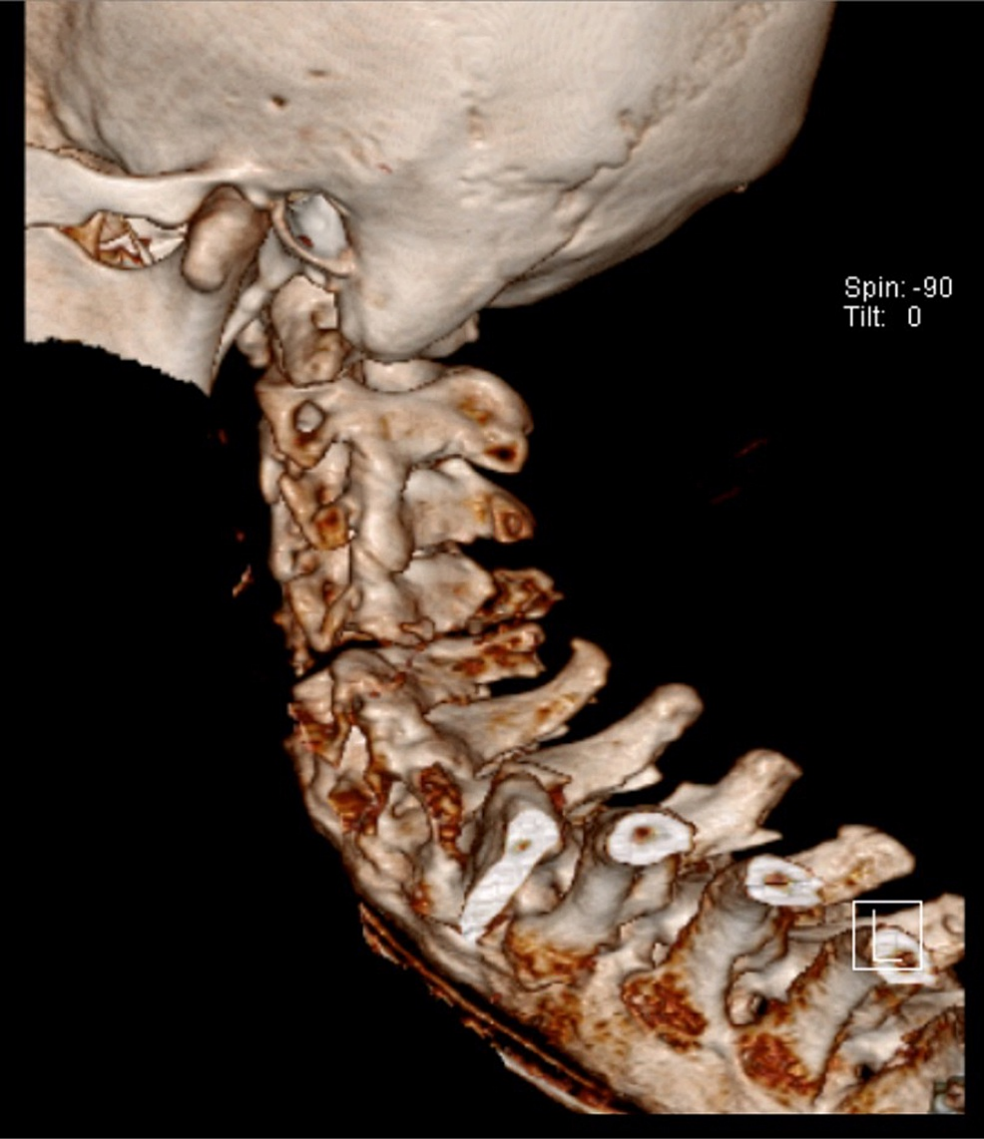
Cervical CT scan with 3D reconstruction revealing fracture-dislocation at C4-5
